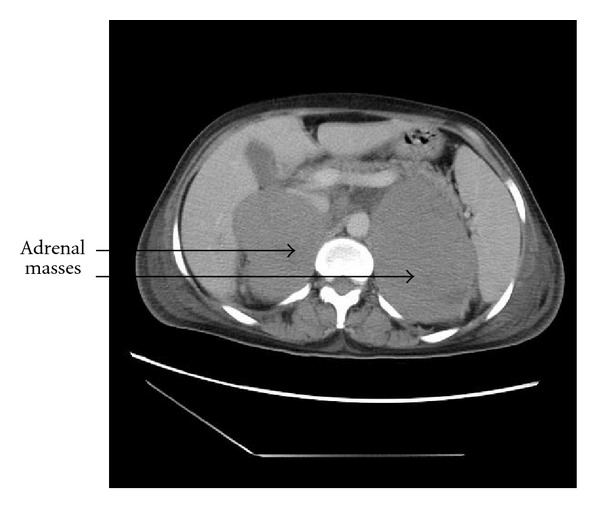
A slight contrast enhancement of the CT scan demonstrated an increase of the volume of adrenal masses.
